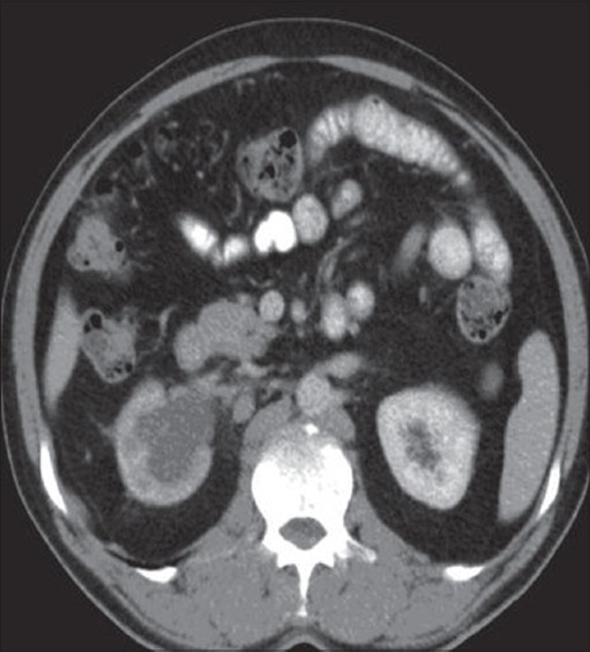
CT showing right hydronephrosis with a small pelvic lesion
